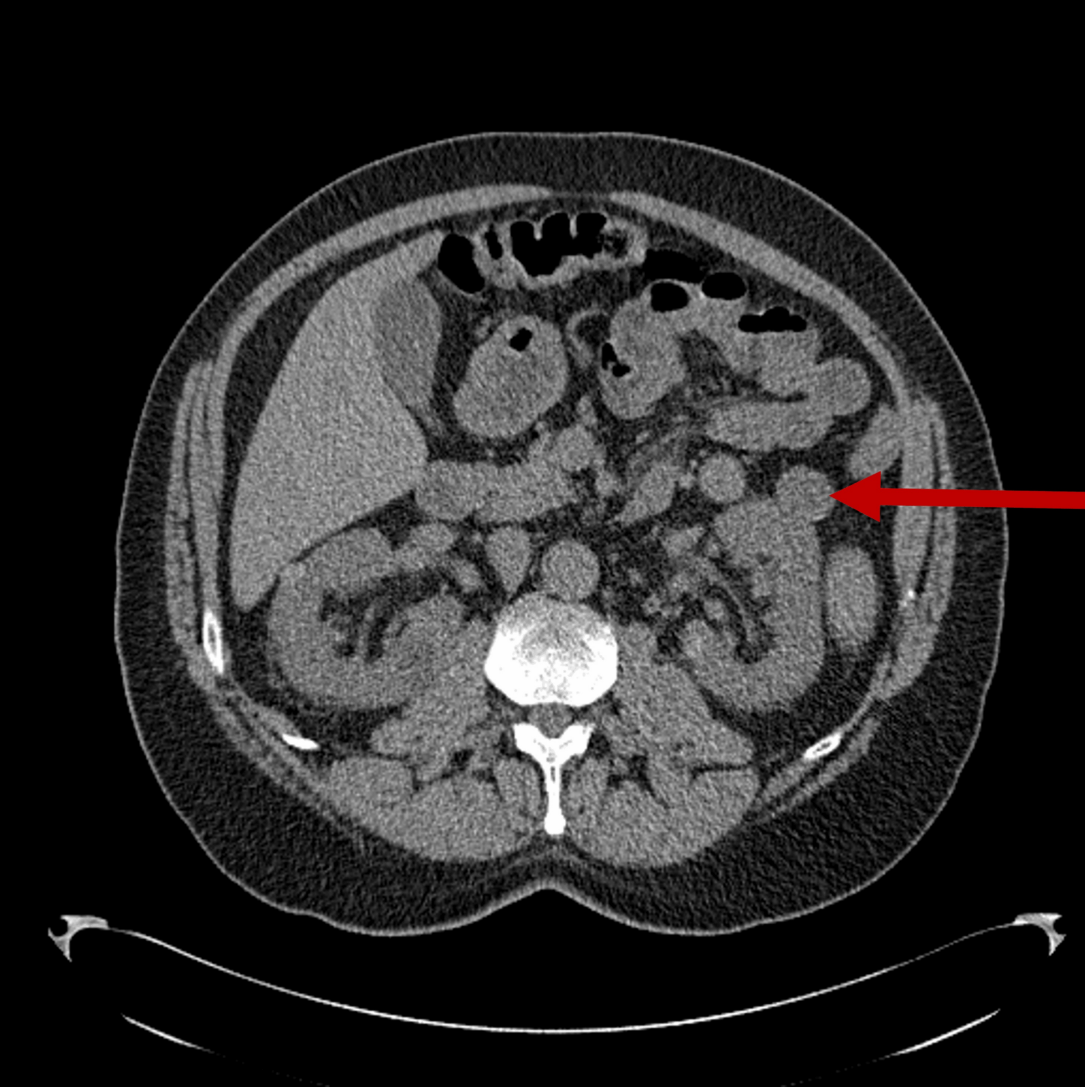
CT of the thorax in September 2020. A CT of the thorax done in September 2020 for lung cancer screening detected an initial renal mass on the left (red arrow).
